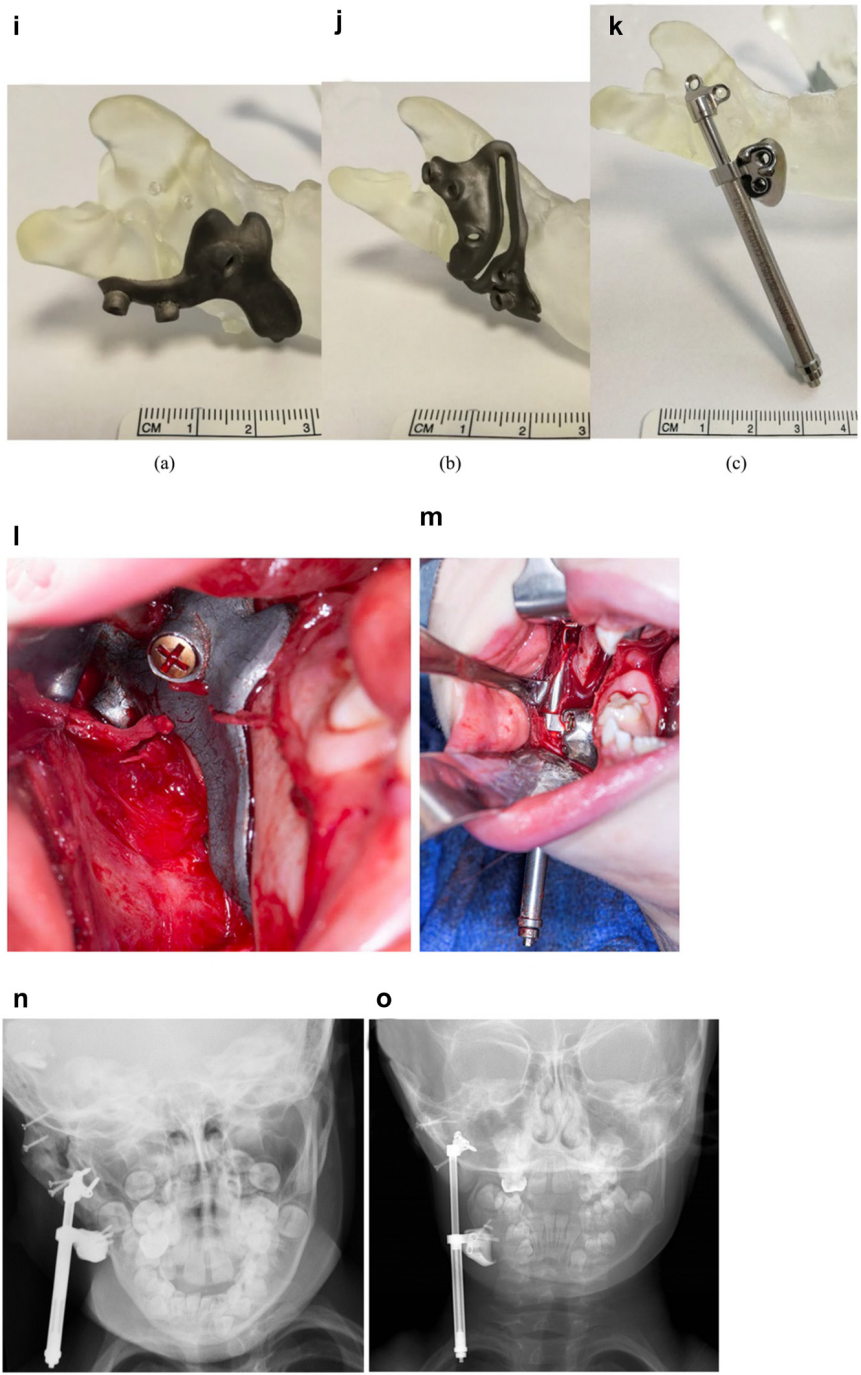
Planning for DO to vertically lengthen the mandibular ramus when the ramus is deficient laterally. (i–k) The 3D printed guides, spacer and distractor. (l and m) During surgery. (n and o) PA radiographs of the start and end points of distraction.
